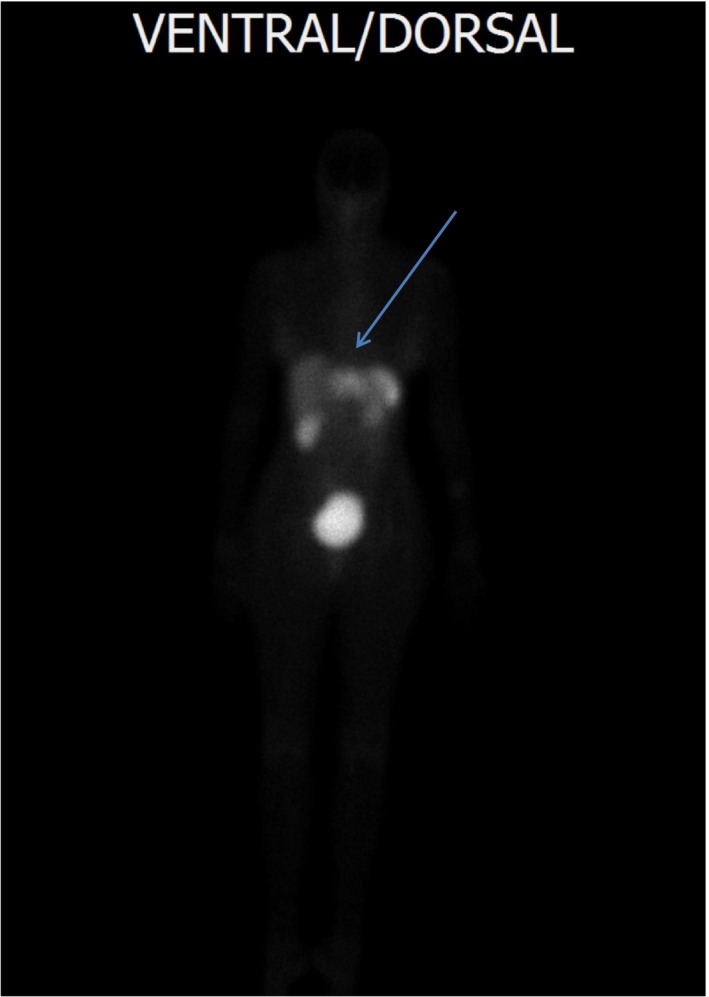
Indium‐111 octreotide scintigraphy. Indium‐111 octreotide scintigraphy of patient 2 revealing increased activity in left hepatic lobe (arrow)
